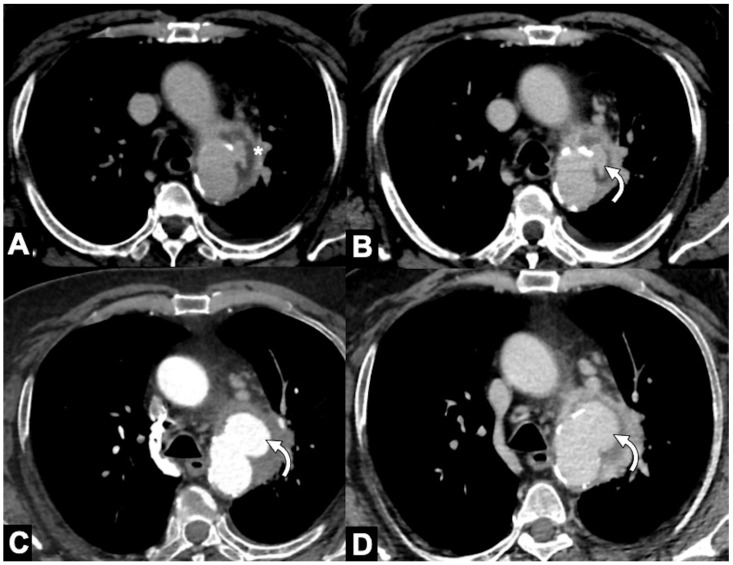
CTA of aortitis and periaortitis by Salmonella species. A 68-year-old man with chills, high temperature, and upper back pain radiating to anterior chest. (A) Unenhanced CTA axial image shows marginal periaortic tissue (asterisk) at aortic isthmus level. (B) Axial CTA late phase image also shows a focal outpouching (curved arrow) of contrast material emanating from the isthmus. (C,D) Axial arterial and delayed-phase CTA images obtained 7 days later show that contrast outpouching was considerably larger (curved arrows), a finding referable to rapidly growing focal mycotic pseudoaneurysm, and the patient underwent endovascular aortic repair and intravenous antibiotic therapy.
